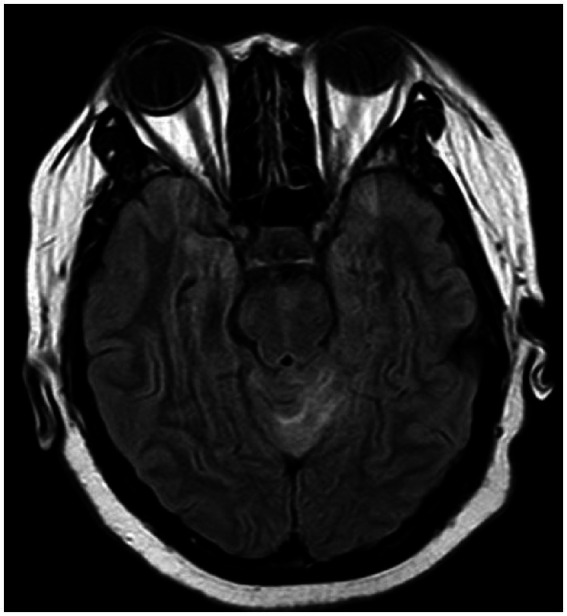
Patchy hyperintensities on T2-weighted FLAIR imaging in the left cerebellar hemisphere and adjacent vermis, suggestive of viral encephalitis.
